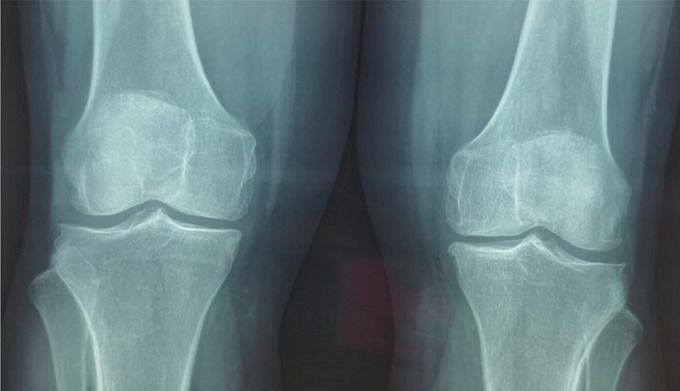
X-ray of knees showing widening of the joint space and bony hypertrophy of the condylar shells
